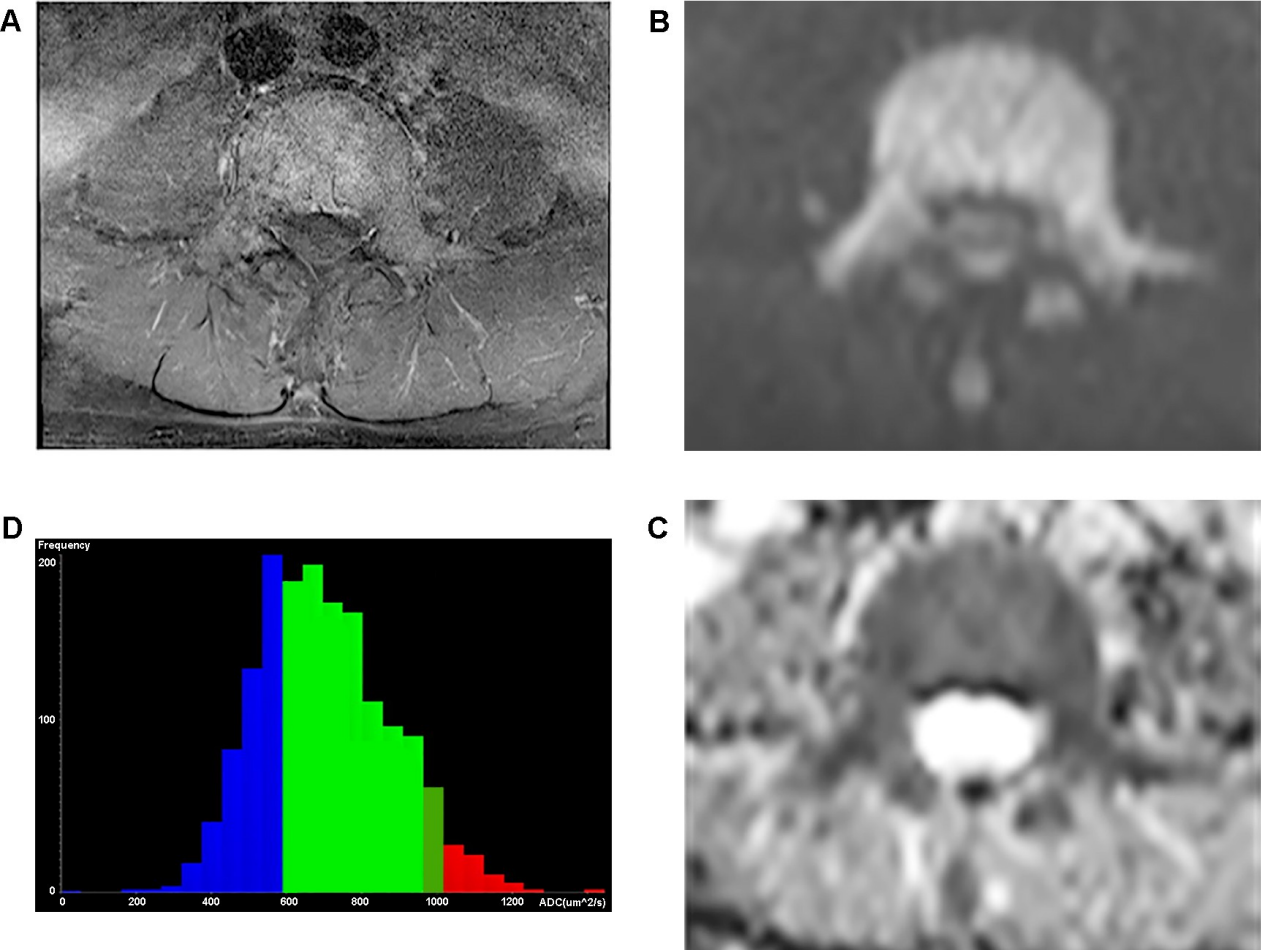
Representative case of multiple myeloma. A 63-year-old man with multiple myeloma. (a) Axial fat-suppressed contrast-enhanced T1-weighted image shows diffuse enhancement in lumbar spine. (b) Axial DW image (b = 800 sec/mm^2^) at the same level reveals diffuse high signals throughout the lesion. (c) Corresponding axial ADC map (b = 0 and 800 sec/mm^2^) shows diffuse low signal intensity. Median value of ADCav was 617 ± 77 μm^2^/sec, ADCmin was 550 ± 75 μm^2^/sec. (d) In the corresponding ADC histogram of whole tumor volume, mean value of ADCvol was 700 μm^2^/sec and SDvol was 178. Interpretation was correct in both readers.
